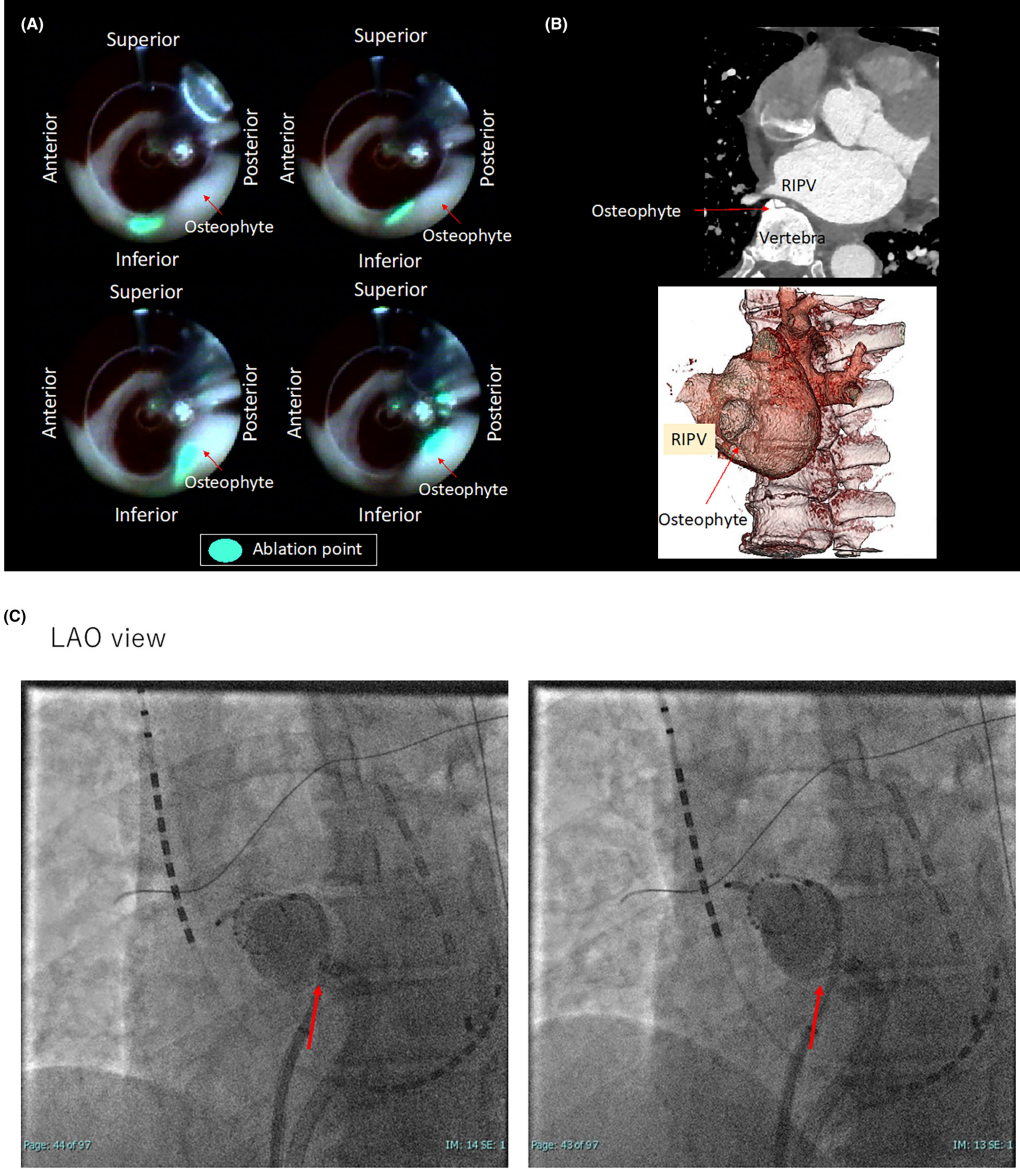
(A) Endoscopic view of the vertebra compressing the left atrial posterior wall. (B) Enhanced computed tomography (axial, 3D) showed the osteophytes of the vertebra pressing against the left atrial posterior wall. (C) Fluoroscopy in left anterior oblique (LAO) view showed the osteophytes of the vertebra pressing against the left atrial posterior wall during respiratory movements during the right inferior pulmonary vein ablation. LAO, left anterior oblique; RIPV, right inferior pulmonary vein.
